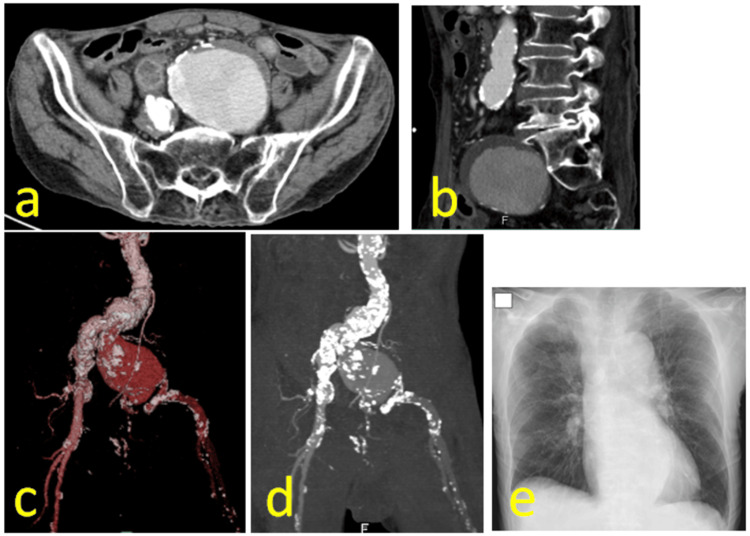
Preoperative findings (a) Contrast-enhanced CT showing a 69 mm left CIAA containing a mural thrombus. (b) The aneurysm was compressed by a sharp bone spur in the lumber vertebrae. (c) The abdominal aortic aneurysm measured 33 mm in diameter, and the right CIAA measured 29 mm. (d) Extensive calcification was observed from the abdominal aorta to both EIA. (e) Chest radiograph showing decreased transparency in the lung fields. CIAAs, common iliac artery aneurysms; EIA external iliac arteries; CT, computed tomography
